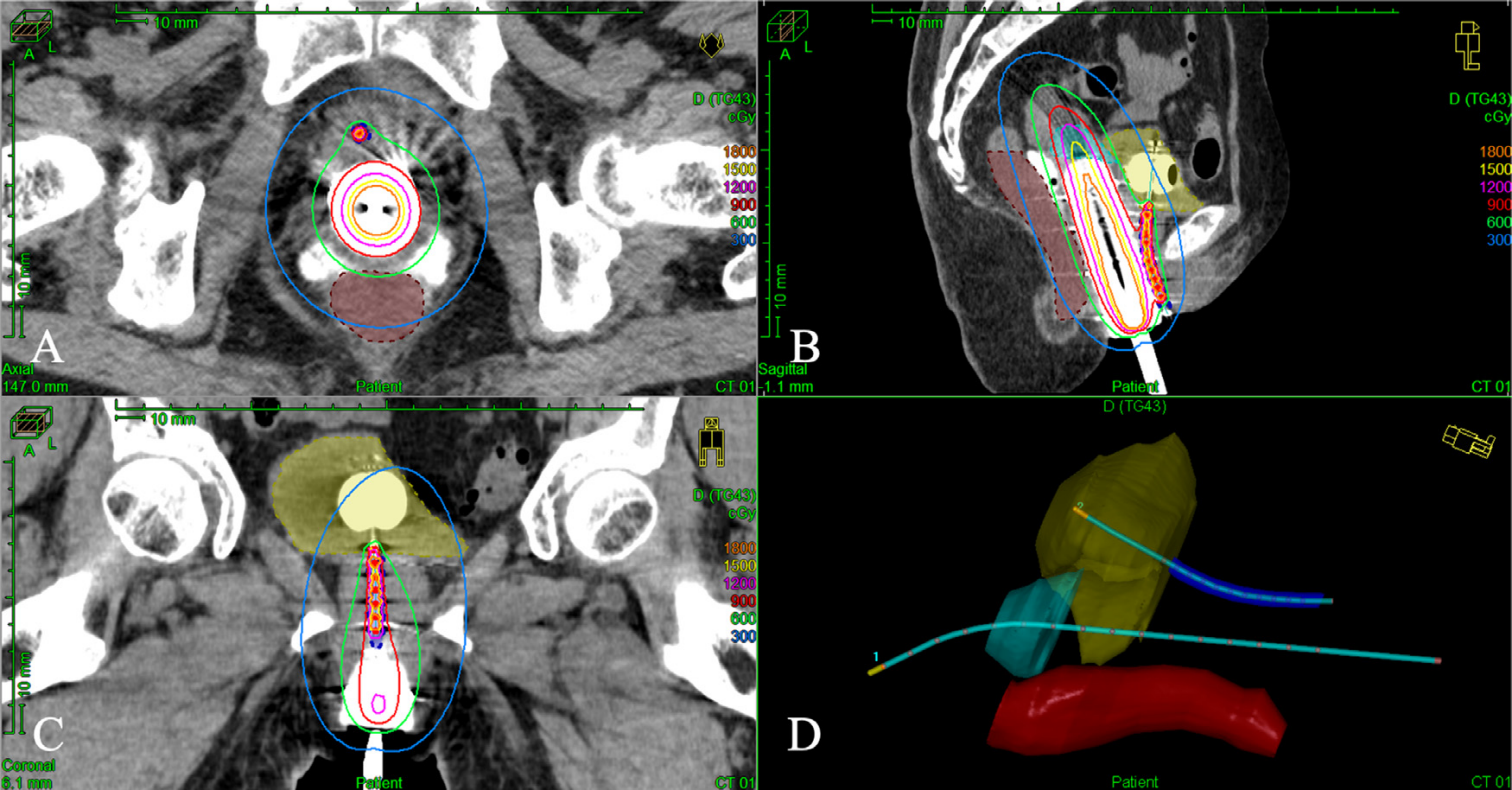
Dose distribution and applicator reconstruction of computed tomography (CT)-based image guided brachytherapy. (A) Axial view, (B) sagittal view, and (C) coronal view of treatment planning. (D) Three-dimensional image of the applicator reconstruction. Light blue shade, clinical target volume of cervix; yellow shade, bladder; brown shade, rectum; blue shade, urethra. Green line represents 100% (6 Gy) isodose line. The red line represents the 150% (9 Gy) isodose line.
